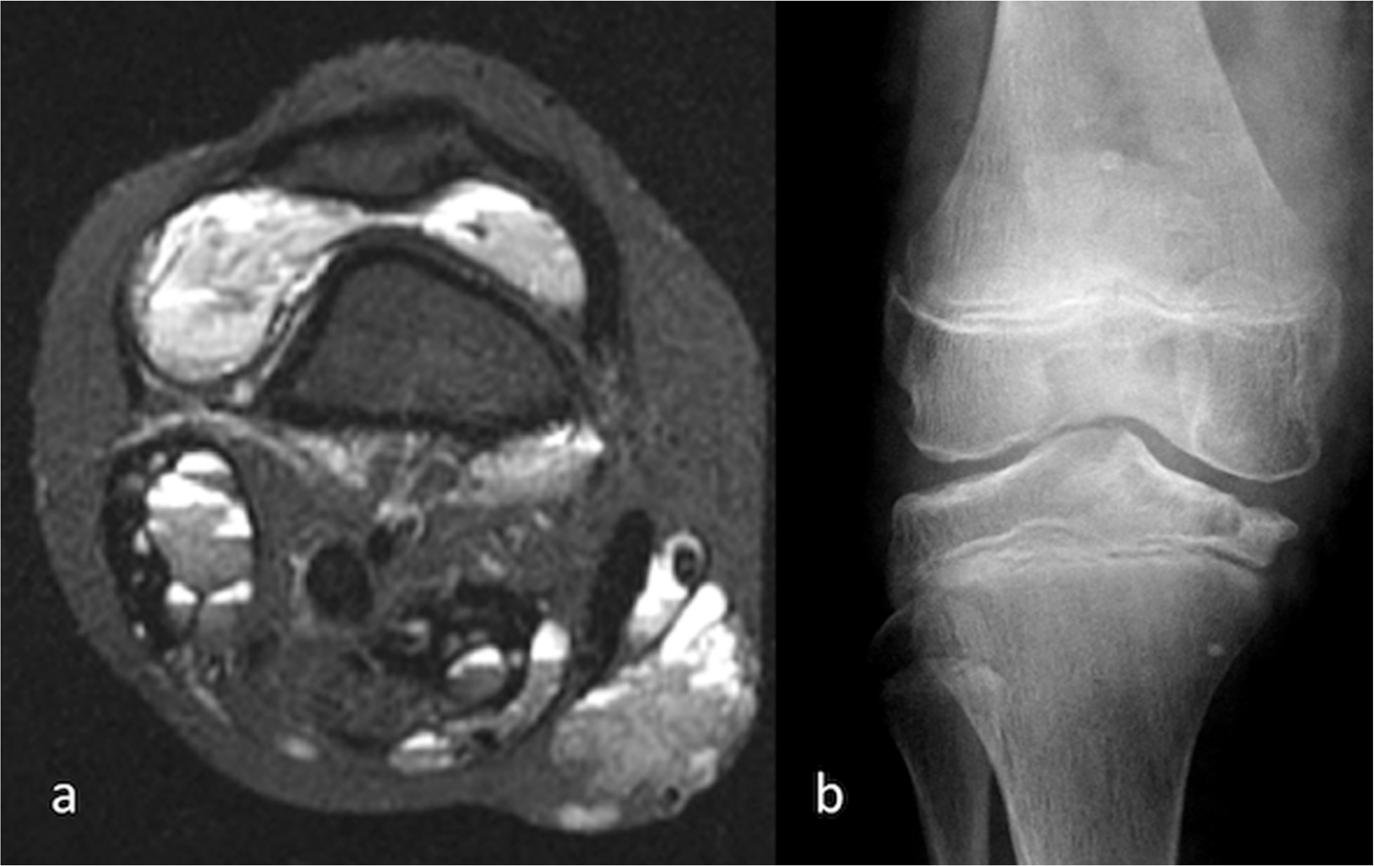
Venous malformation (VM) of the knee in a 10 year old female. a Axial T2 weighted MR image shows VM within the soft tissues around the knee and within the knee joint itself; b AP radiograph confirms severe articular damage to the knee joint. Soft tissue thickening and phleboliths are also noted
